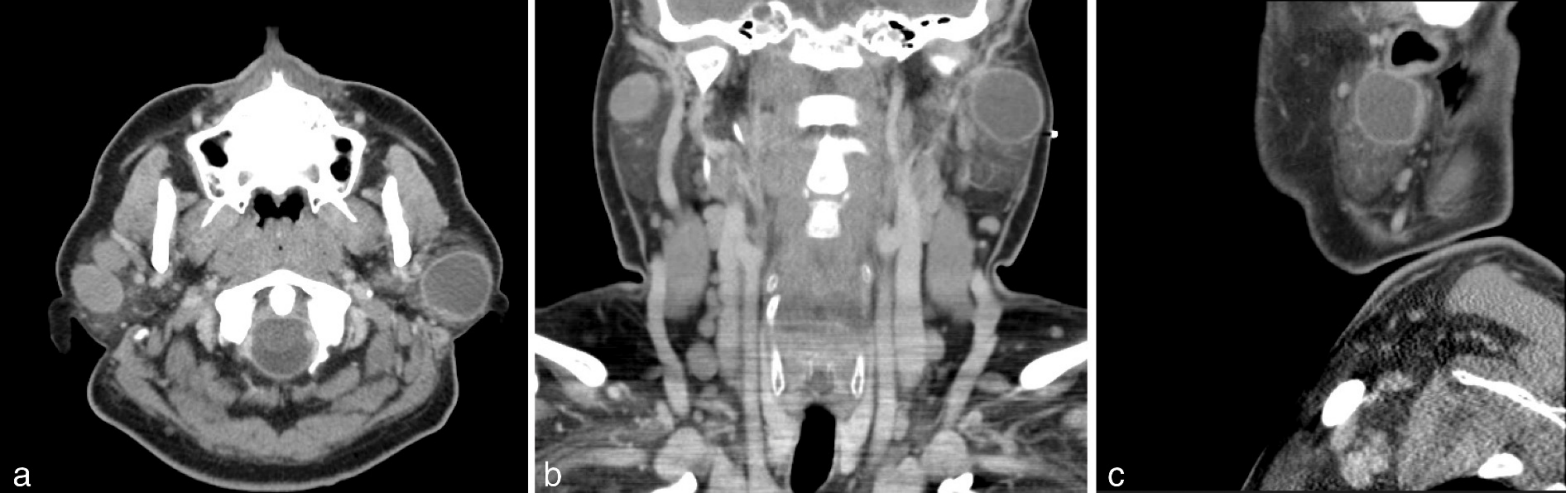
(a) Axial contrast-enhanced CT scan of the neck demonstrating bilateral parotid gland masses. Dominant ring-enhancing hypodense lesion of the left parotid gland measures 3.0 × 2.9 cm. (b) Coronally reconstructed CT scan with a prominent left cystic parotid mass and a smaller dense lesion of the right parotid gland. Few enlarged level 2 lymph nodes are noted bilaterally, ranging from 1.0 to 2.4 cm. Numerous non-pathologically enlarged jugular chain lymph nodes are visualized. (c) CT scan of the neck in sagittal reconstruction revealing a hypodense lesion with rim enhancement within the left superficial parotid gland.
